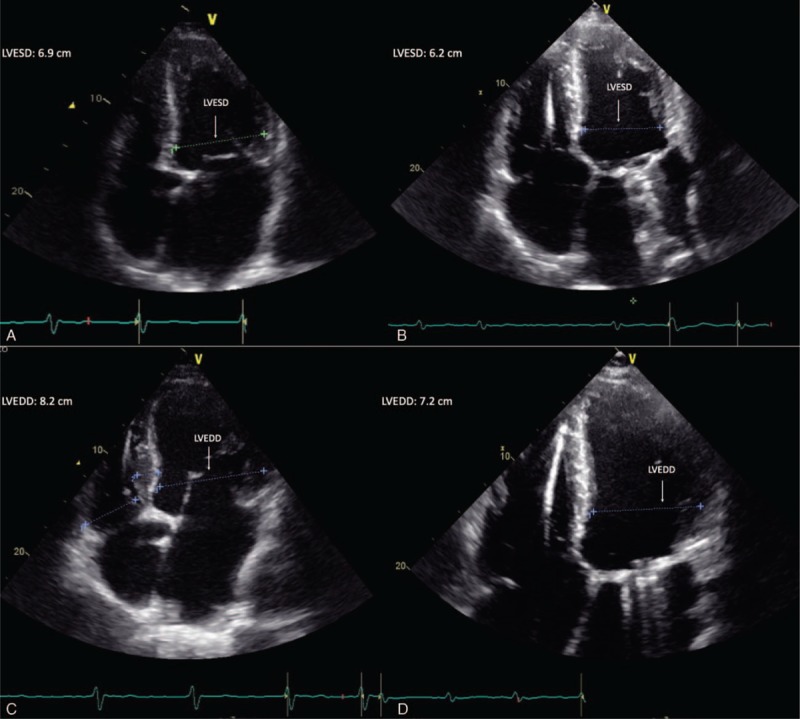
Echocardiographic features before (A, C) and after (B, D) 9 months of sacubitril/valsartan administration. LVEDD = left ventricle end-diastolic diameter, LVESD = left ventricle end-systolic diameter.
